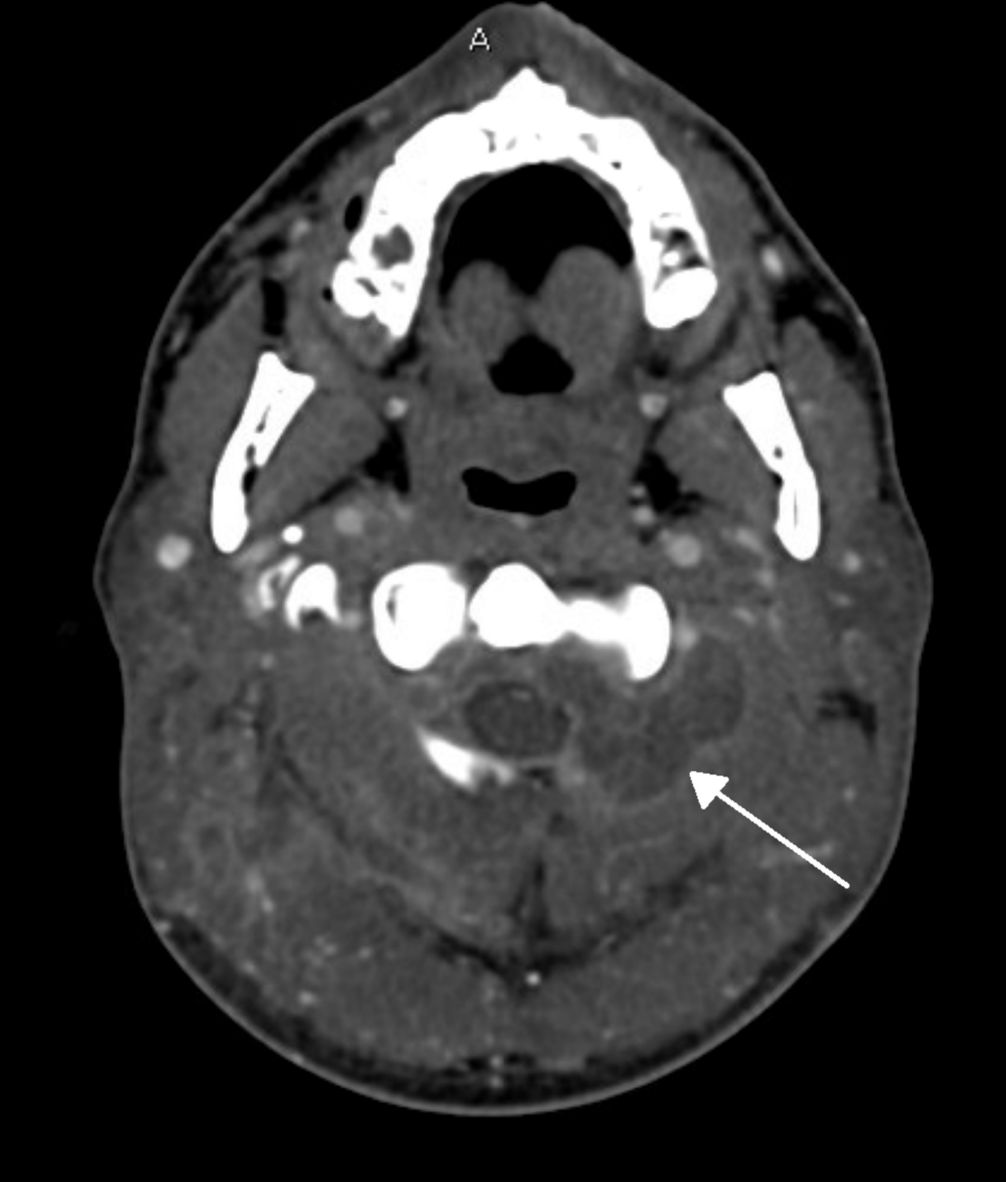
Axial CT angiography of the neck showing multiloculated abscess Image showing multiloculated abscess (arrow) extending into the left C1-C2 neuroforamina causing partial effacement of the anterolateral thecal sac.
